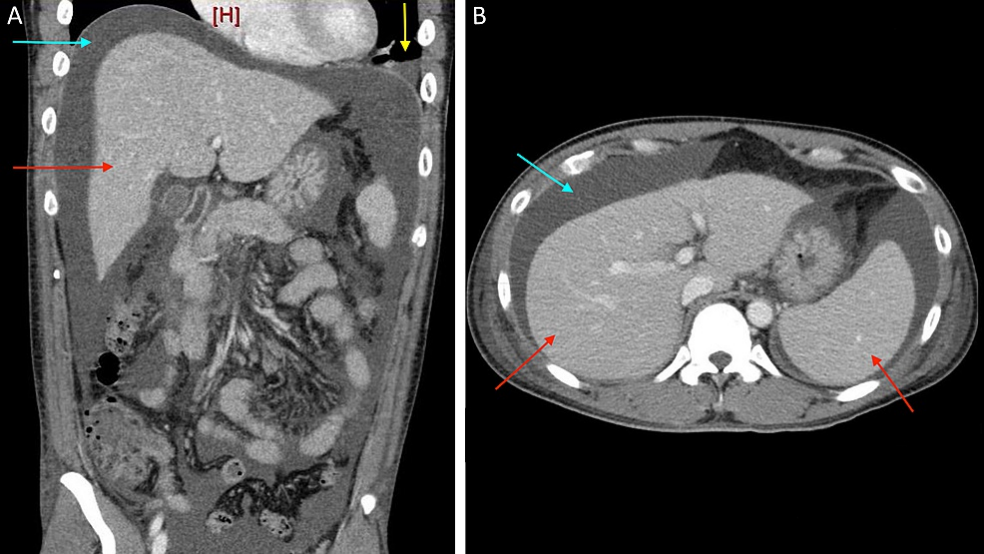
CT of the abdomen and pelvis. Coronal plane (A) and transverse plane (B) show a small pleural effusion (yellow), ascites (blue), and hepatosplenomegaly (red). No peritoneal lesions were identified.
